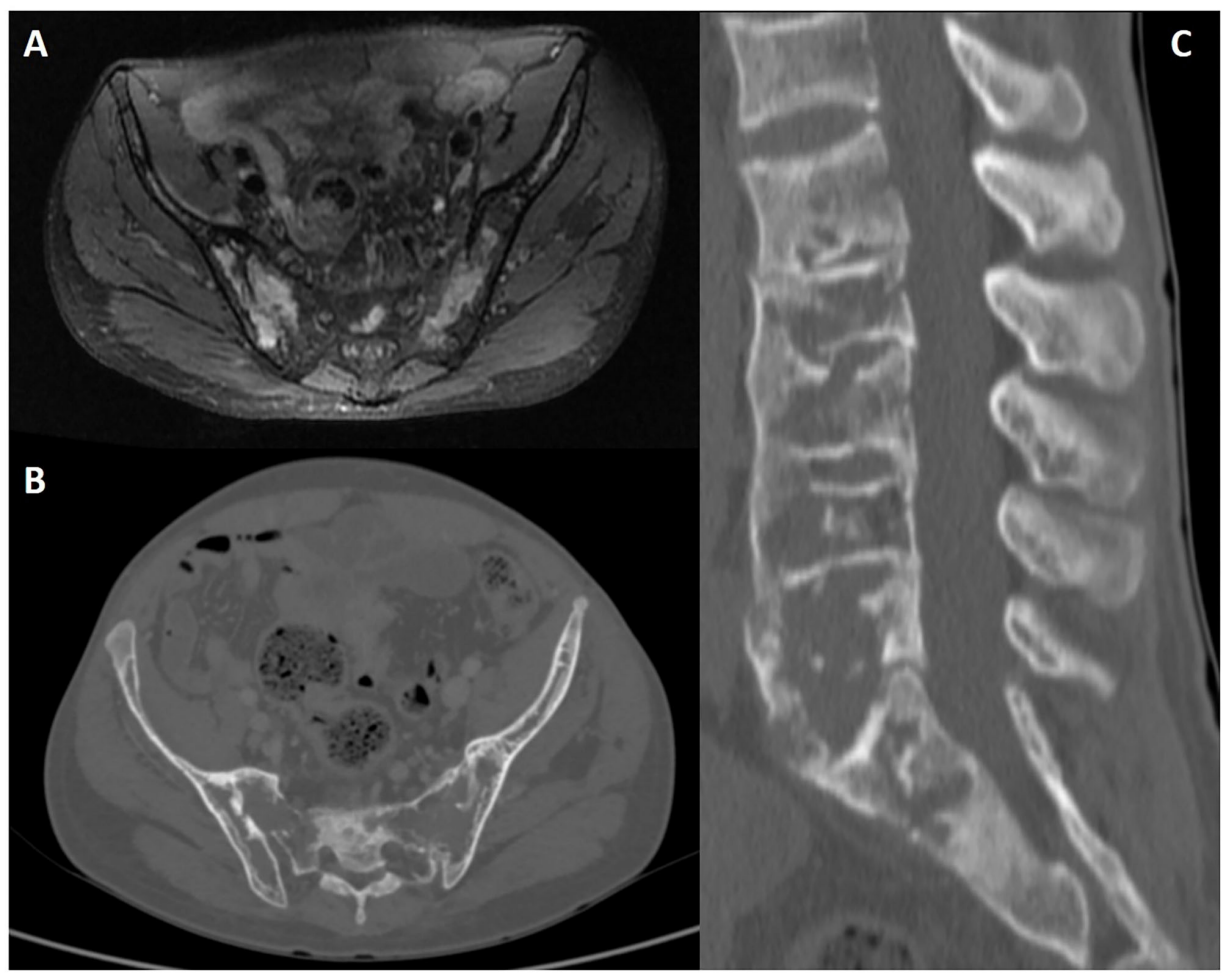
Fat-saturated PD (A) MRI sequence, CT axial slice (B), and sagittal lumbar spine reconstruction (C) of a 31-year-old male patient with Sickle cell disease and extensive bone marrow changes causing widening of the medullary spaces and thinning of cortical bone.
